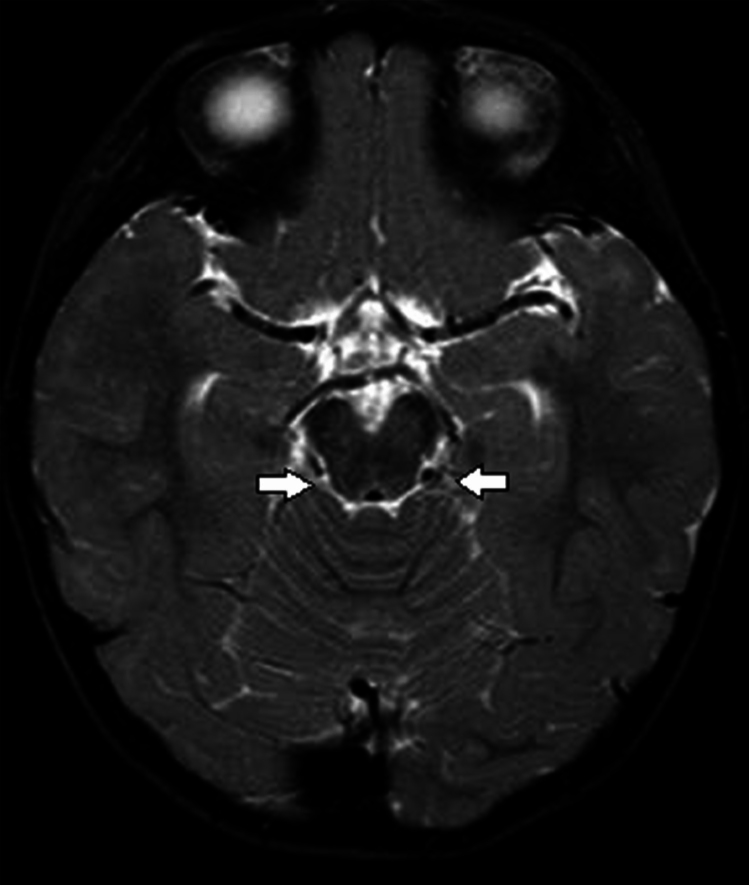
Magnetic resonance imaging of the brain of proband III:2. The thin linear structure coursing obliquely from the left pontomedullary region in the left pontine cistern suggests the left abducens nerve, which was absent in the right side.
